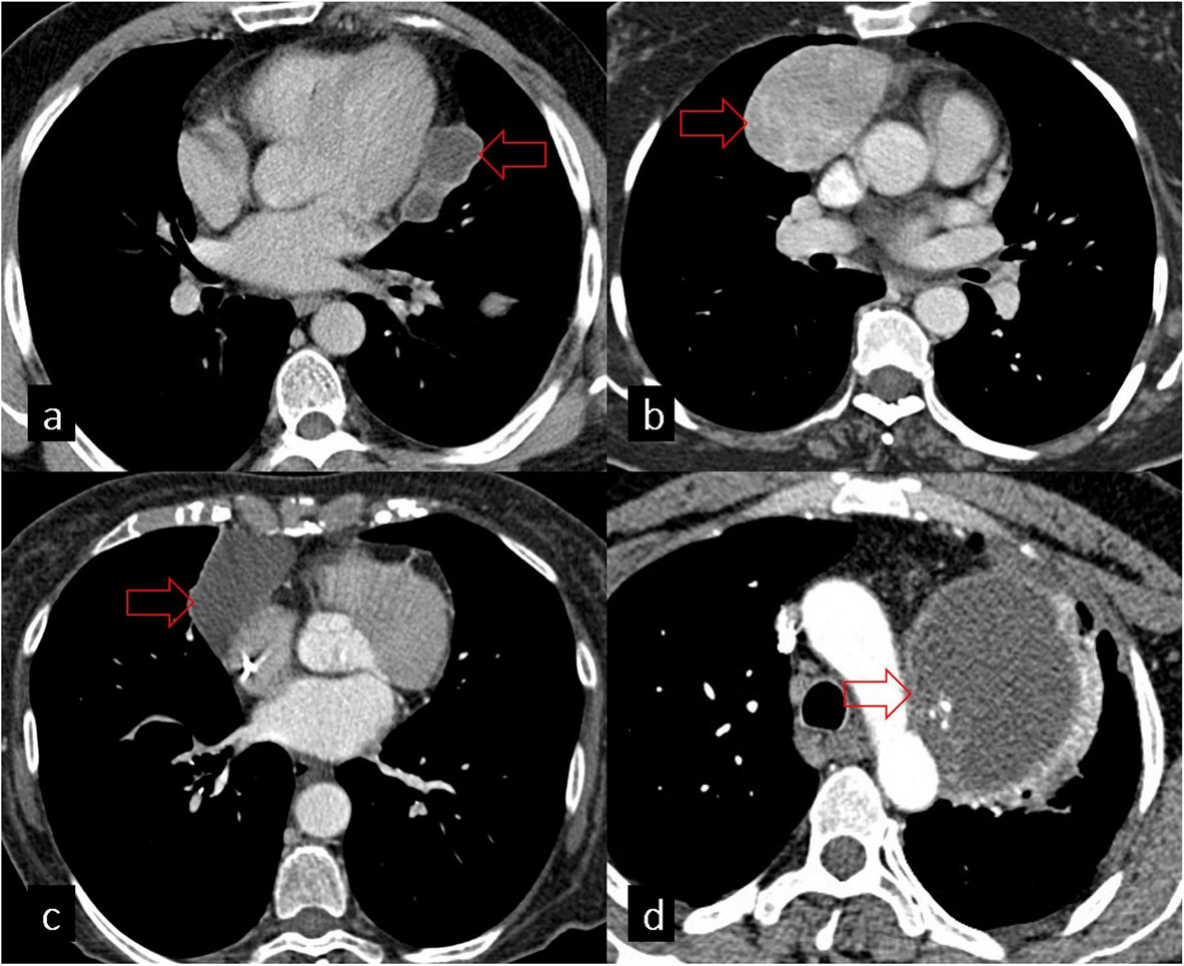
a Axial CT image shows a cystic lesion in the mediastinum, adjacent to the left ventricle, pathologically proven as a hydatid cyst (red arrow). b, c, d Differential diagnosis of mediastinal hydatid cysts: thymoma (b), pericardial cyst (c), and teratoma (d)
